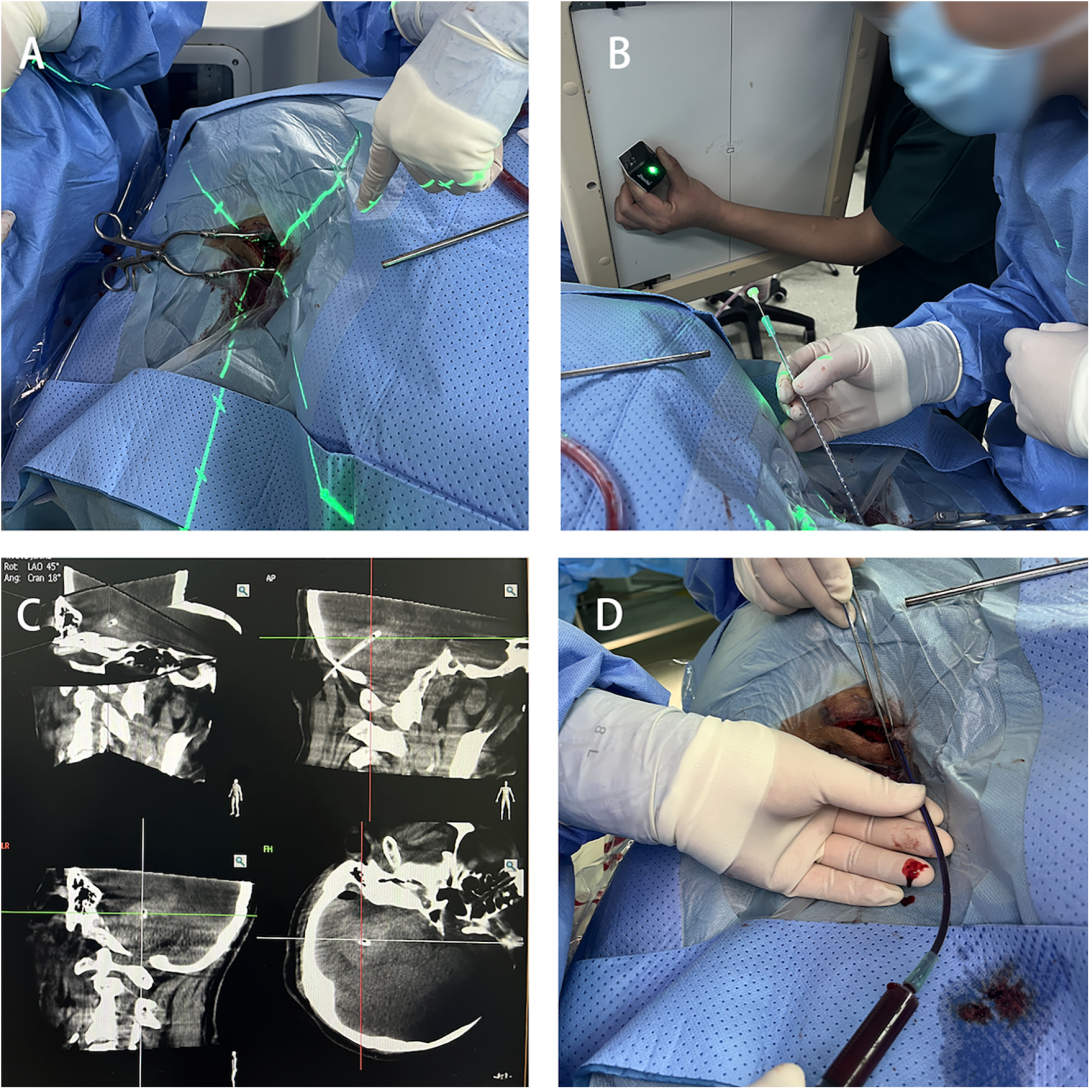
Hematoma puncture. (A) Laser crosshair aligning with the bone hole position. (B) Puncture drainage tube positioned at the center of the laser crosshair. (C) C-arm CT scan confirming the catheter position. (D) Aspiration of part of the hematoma for decompression.
